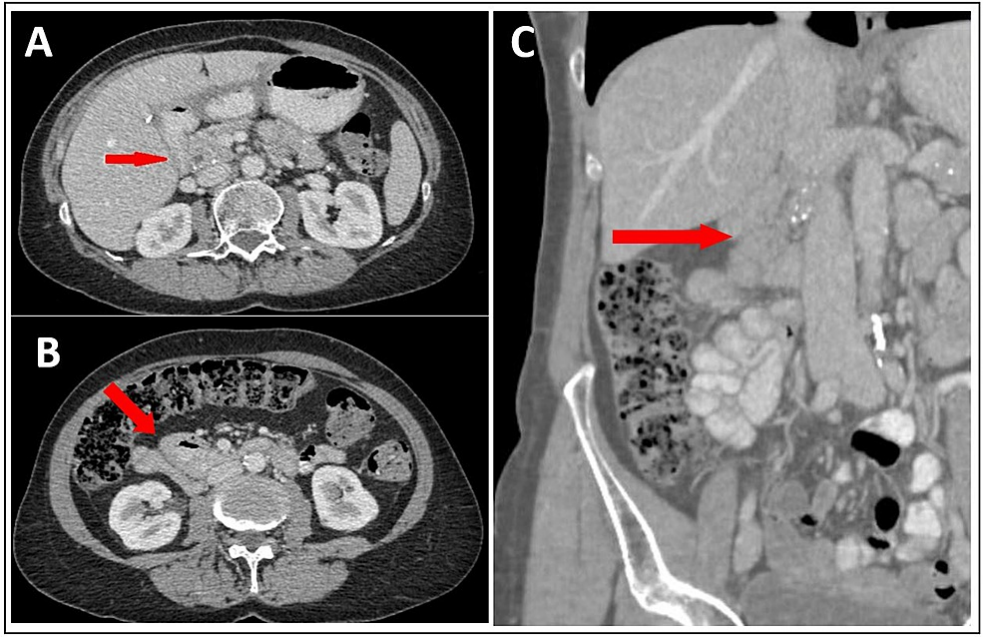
Postoperative CT scan Axial (A) and coronal (C) CT scan slides on postoperative day 13 showing no relapse of the abscess (red arrows) and no anastomotic leak (B, red arrow)
